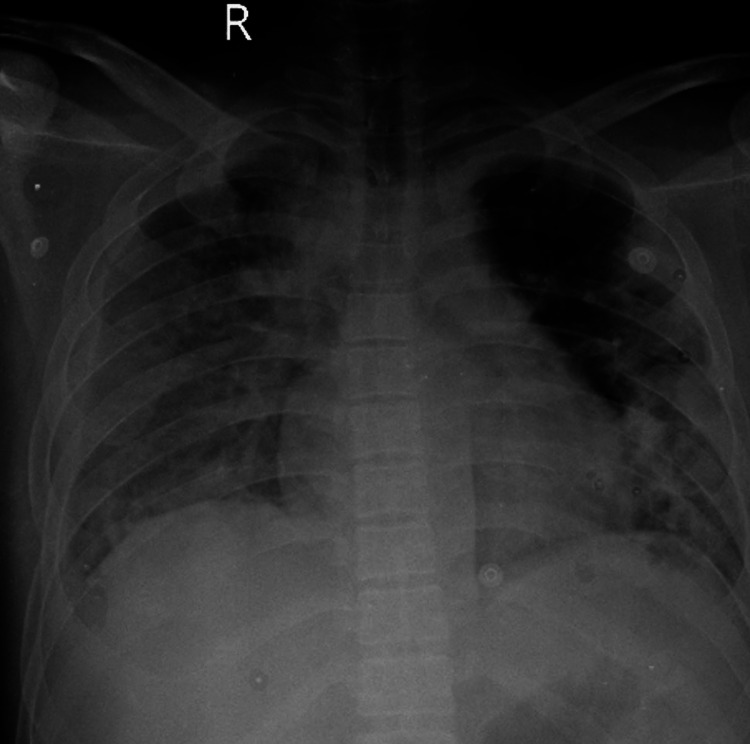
Chest x-ray posteroanterior (PA) view on admission suggestive of bilateral pulmonary infiltrates with right-sided pleural effusion and haziness in the left lower zone
